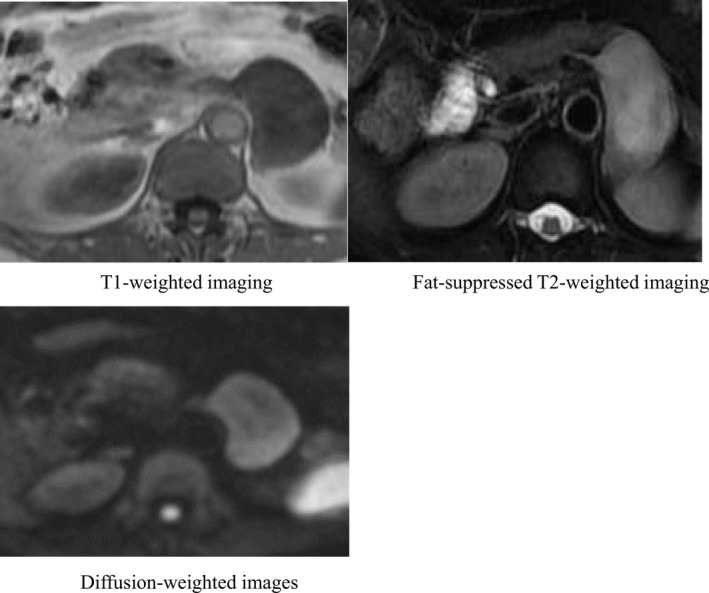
Abdominal contrast‐enhanced magnetic resonance images. Low signal on T1‐weighted imaging, faint and heterogenous high signal on T2‐weighted imaging, and faint high signal on diffusion‐weighted images. T1‐weighted imaging, Fat‐suppressed T2‐weighted imaging, Diffusion‐weighted images
